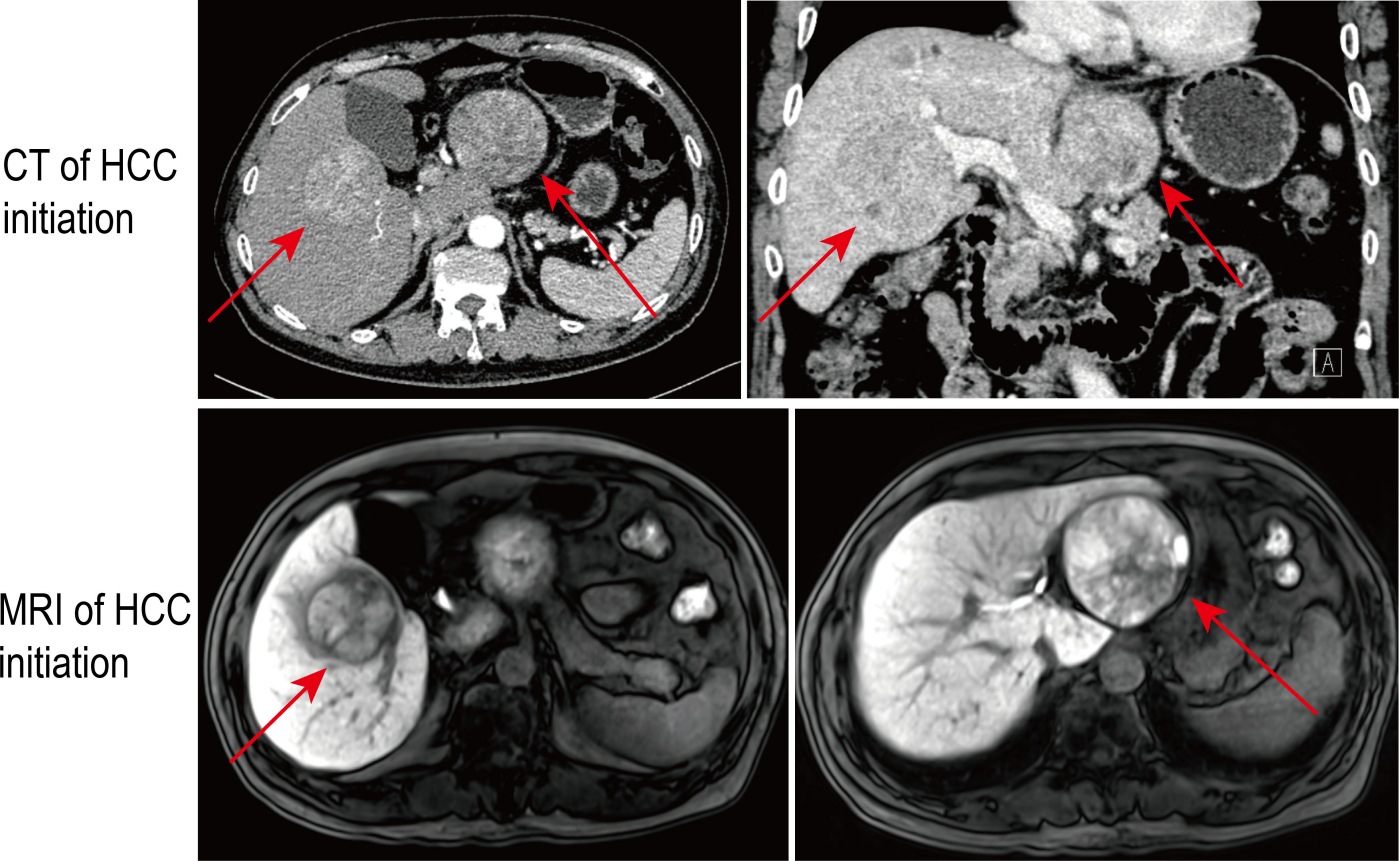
The multiphase liver protocol computed tomography (CT) and gadoxetate disodium–enhanced MRI scan demonstrated the bulky bilobar liver masses with a characteristic radiological appearance of hepatocellular carcinoma (HCC) at first presentation. The red arrows indicate the intrahepatic bilobar tumors.
